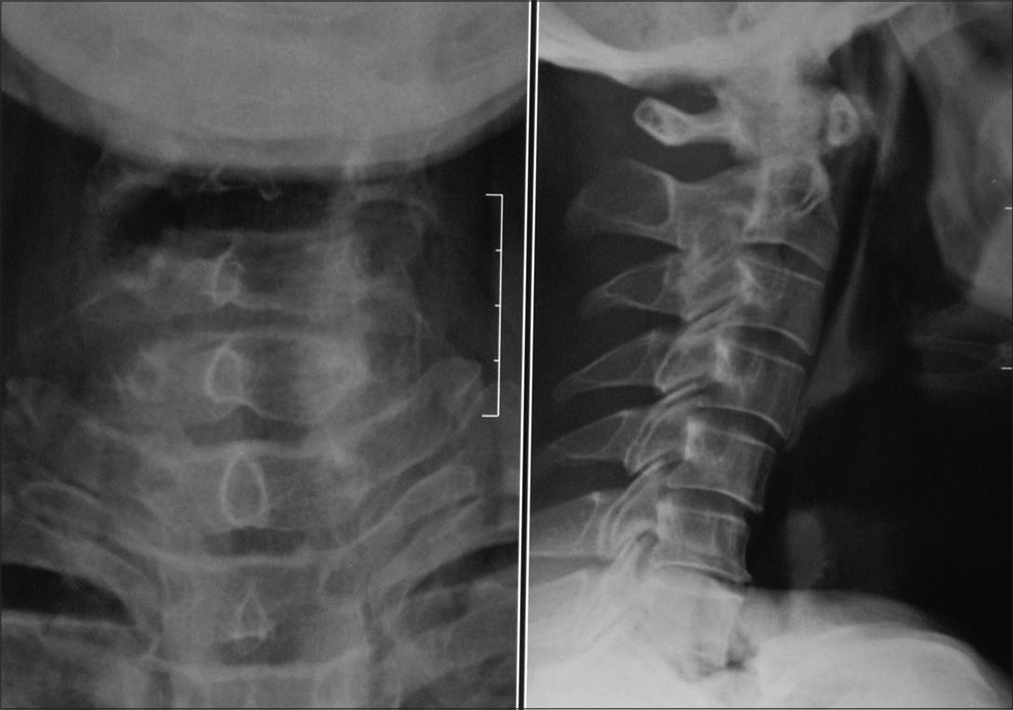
X-ray soft tissue neck showing a transverse air shadow in AP view and below hyoid bone in lateral view at the neck wound
